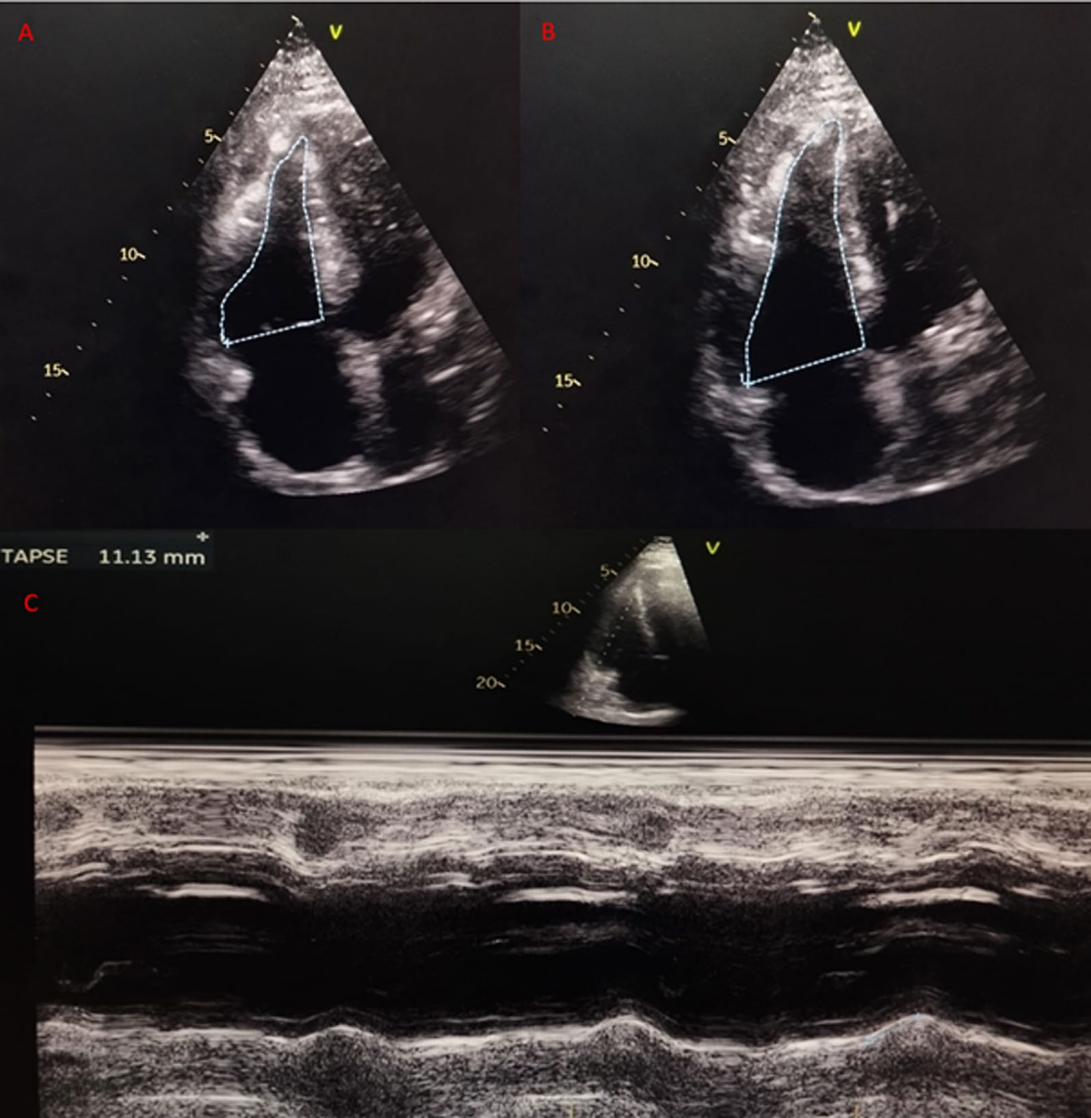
The echocardiography showed (A, B) fractional change area of the right ventricle of 24% and (C) tricuspid annular plane systolic excursion of 11.13 mm
